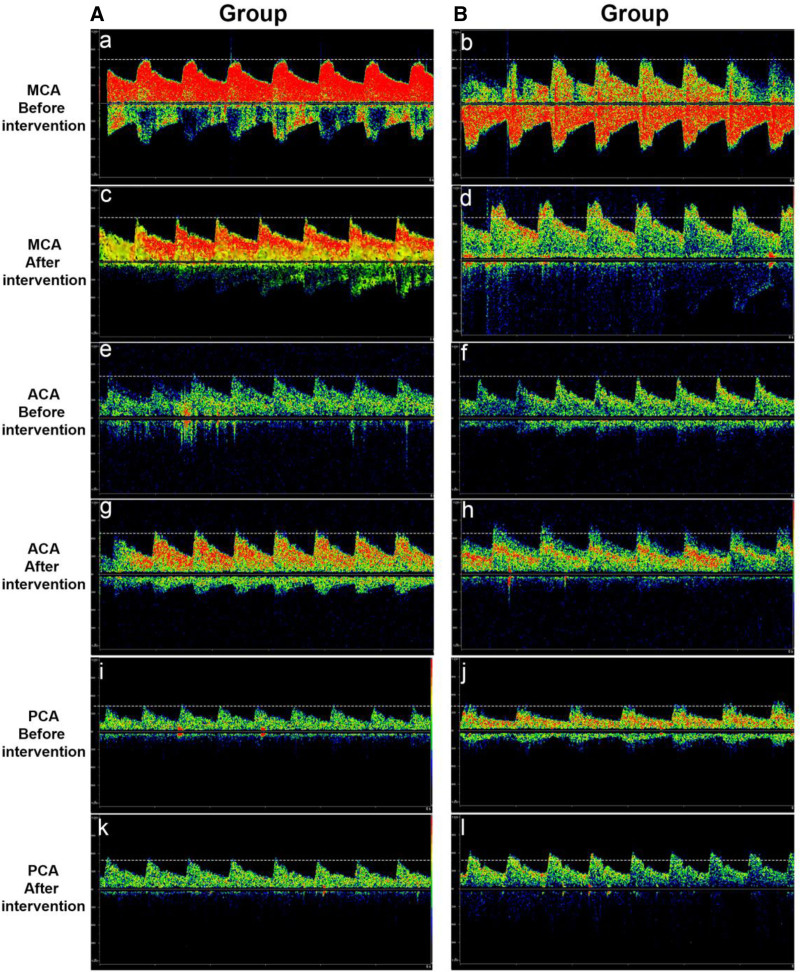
Vascular ultrasound spectrogram: Group A is shown on the left, and Group B on the right. (A and B) Ultrasound images of the middle cerebral artery before treatment; (C and D) posttreatment ultrasound images of the middle cerebral artery; (E and F) Ultrasound images of the anterior cerebral artery before treatment; (G and H) posttreatment ultrasound images of the anterior cerebral artery; (I and J) Ultrasound images of the posterior cerebral artery before treatment; (K and L) Ultrasound images of the posterior cerebral artery after treatment.
